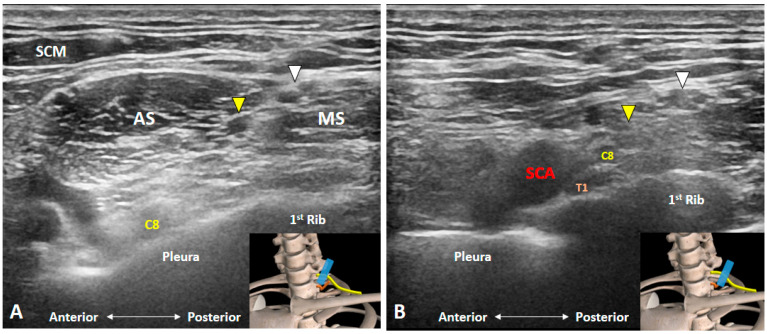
Ultrasound imaging of the C8 and T1 anterior rami. The C8 anterior ramus (A) is seen on top of the pleura at the bottom of the inter-scalene groove. T1 anterior ramus (B) can be visualized distal and lateral to the inter-scalene groove, emerging from the undersurface of the first rib. AS: anterior scalene muscle; MS: middle scalene muscle; SCA: subclavian artery; SCM: sternocleidomastoid muscle. White arrowheads: superior trunk; Yellow arrowheads: middle trunk. The blue rectangles at the bottom-right corners represent the transducer position.
